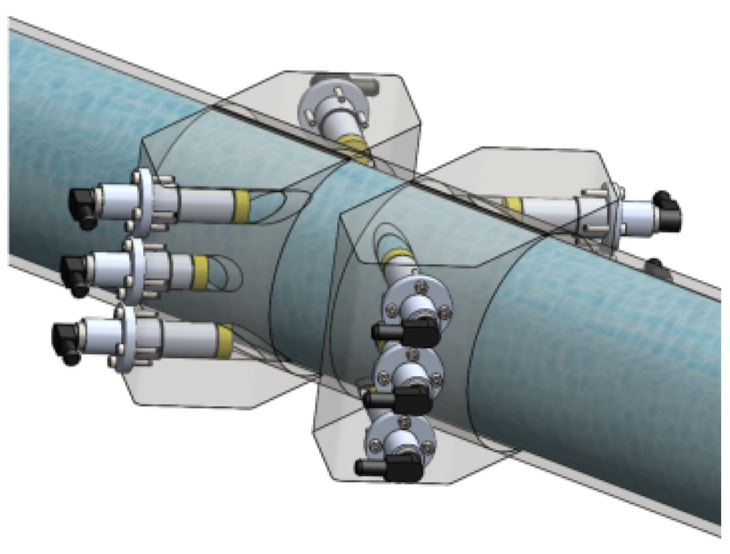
Ultrasonic sensors forming two planes of three trajectories.
